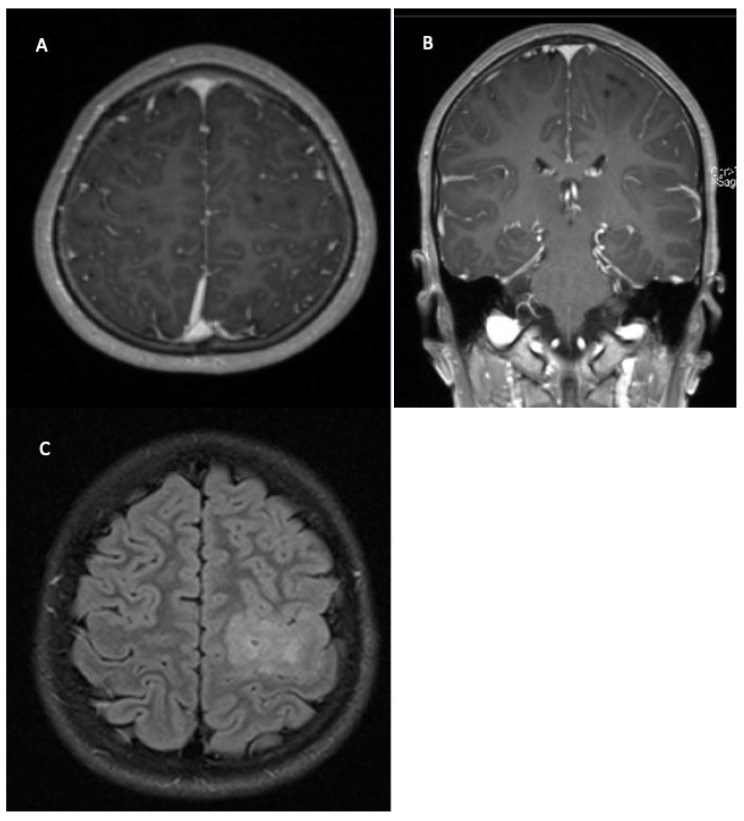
13 year old boy with right hand paresis and initial focal seizure. Contrasted T1 MRI shows non-enhancing cortical tumor (A,B), FLAIR sequence with diffuse cortical lesion (C). Diagnosis: diffuse hemispheric glioma, H3 G34-mutant (WHO grade 4). Therapy: Near total resection, conformal radiation therapy, temozolomide, tumor treating fields (TTF).
